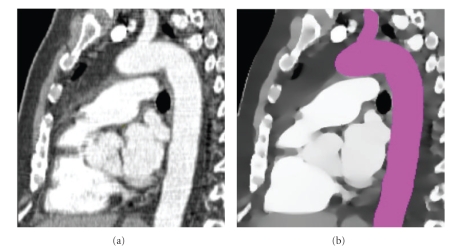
(a) Two-dimensional sagital slice through computer tomography (CT) data of thorax with vascular contrast used in preliminary testing of our algorithm. (b) Mean of means *μ*
